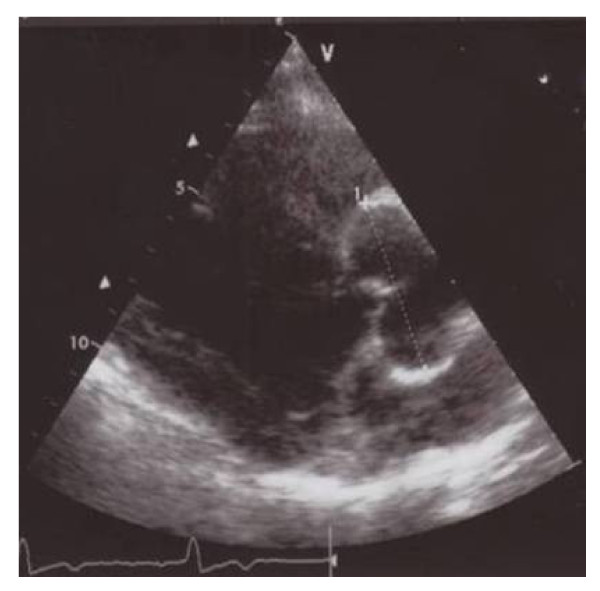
Aortic root dilatation: echocardiography shows aortic root diameter of 47 mm in a 51-year-old male patient with Fabry disease. Courtesy: Pr Olivier DUBOURG and Pr Dominique GERMAIN, University of Versailles - St Quentin en Yvelines (UVSQ), Versailles, France.
